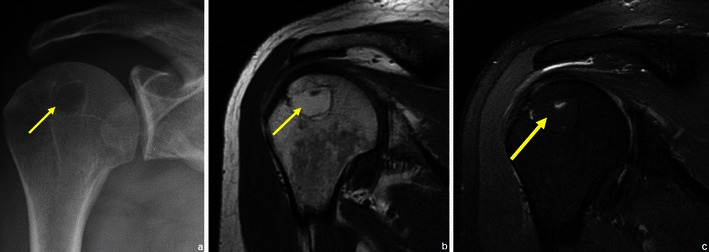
Patient with a humeral lipoma (arrows in a, b and c): frontal radiograph shows a lytic lesion in the head of the humerus. This lesion showed signal intensity similar to that of fat on T1WI (b). The signal was suppressed in T2FS WI (c)
